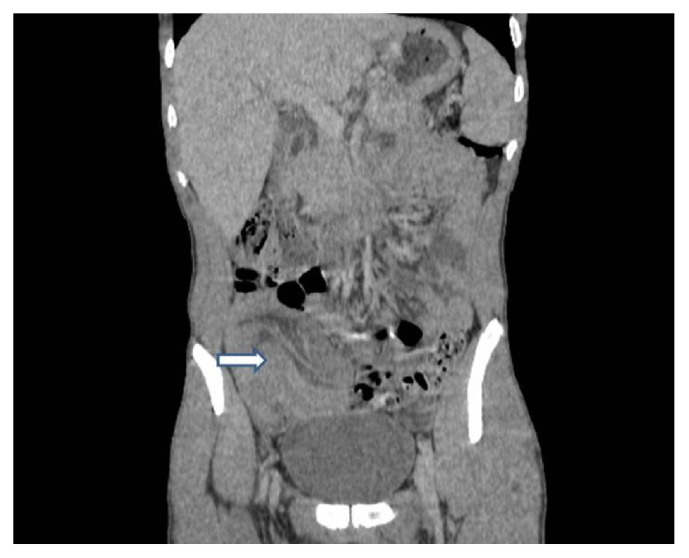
Coronal CT scan of the abdomen and pelvis showing a pseudokidney mass (arrow).
